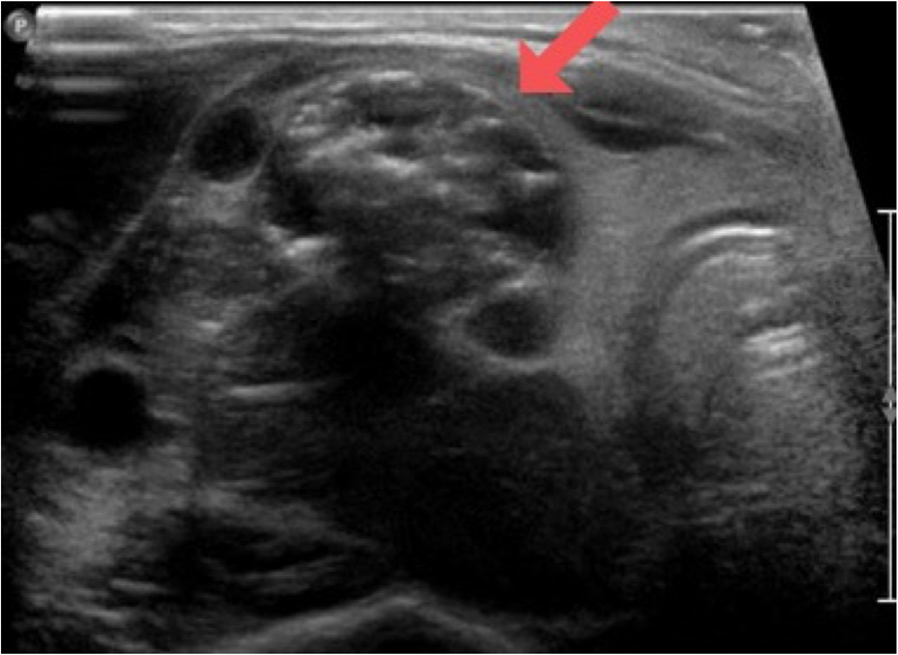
Second ultrasound. Transverse view thyroid ultrasound showing the enlarged right thyroid lobe consisting of multiple solid hypoechoic nodules some with calcifications. The largest nodule in right mid-pole (red arrow) measures 2 × 1.6 × 2.1 cm
